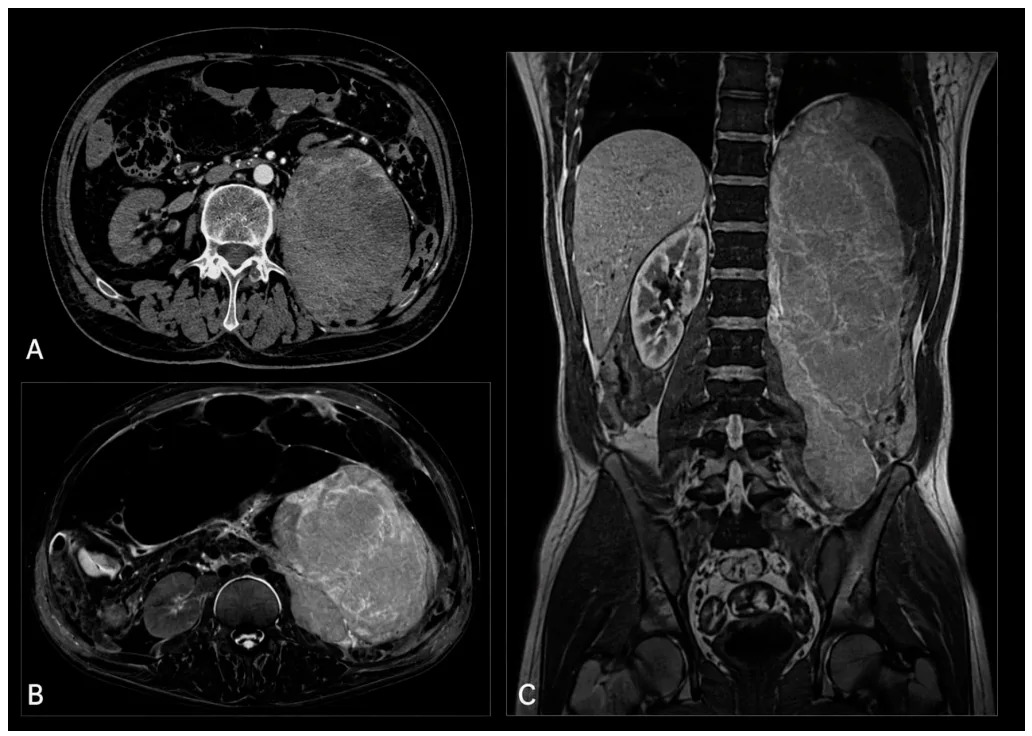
Case 2. A 56-year-old man presented with a several-month history of progressive left-sided low-back and flank pain radiating to the groin and anterior thigh. Axial contrast-enhanced CT (A) and axial T2-weighted MRI (B) demonstrate a large, well-circumscribed left retroperitoneal mass abutting the psoas muscle and the expected course of the lumbar plexus. Coronal T2-weighted MRI (C) depicts its full craniocaudal extent and relationship with the left kidney. Original Image.
